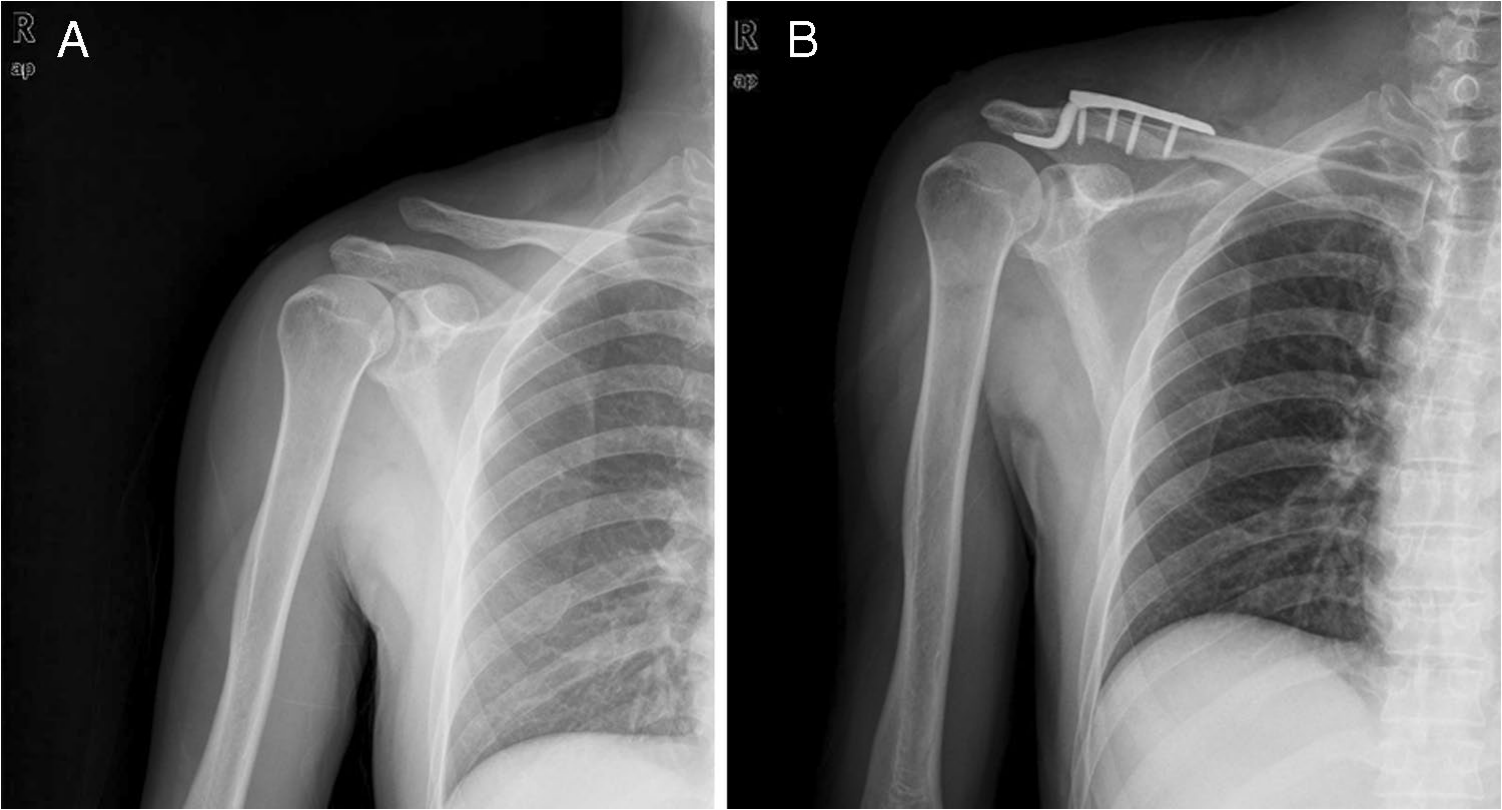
A Pre-operative radiograph showing grade V acromioclavicular dislocation. B Preoperative radiograph showing reduction of dislocation
